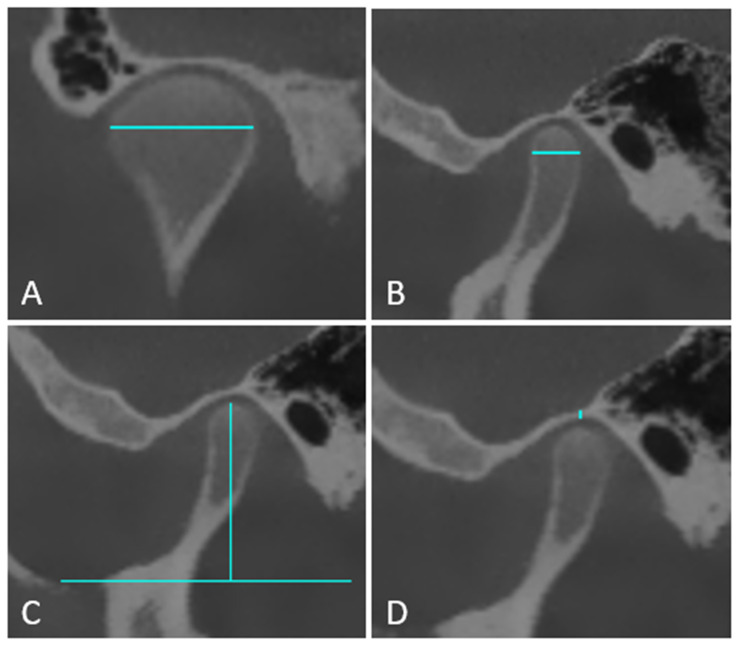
CBCT image demonstrating the measurements of condylar width (A), condylar depth (B), condylar height (C), and glenoid fossa thickness (D).
